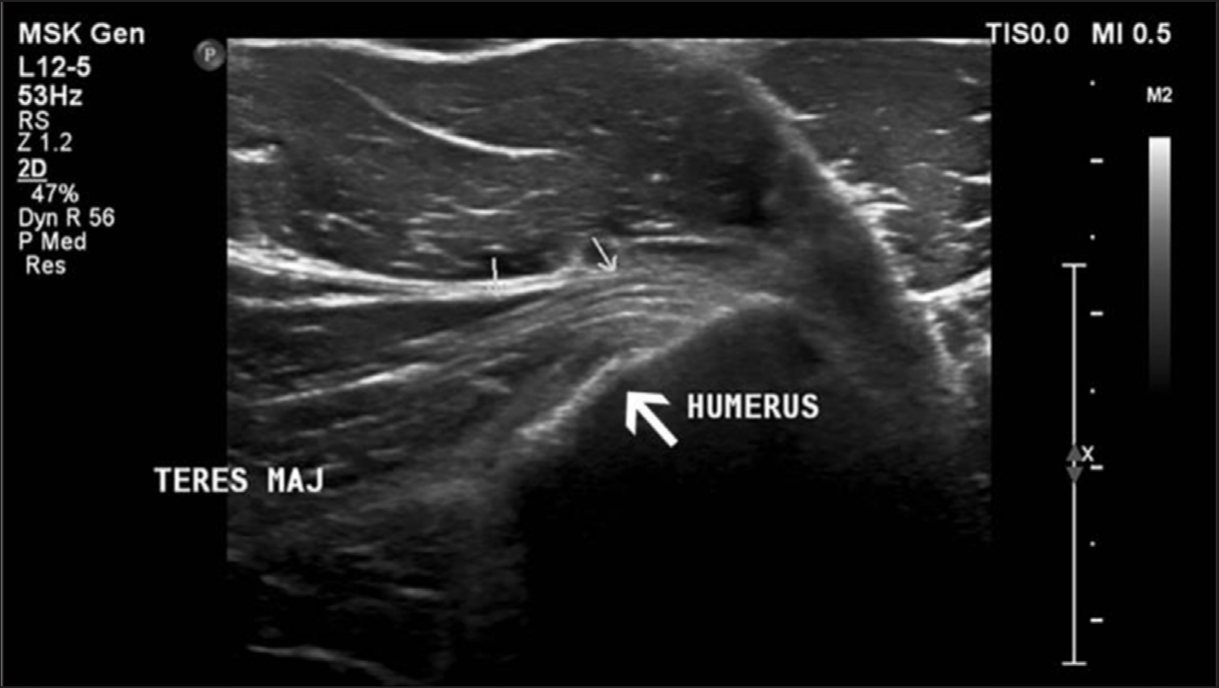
Ultrasound imaging (axial image) reveals an irregular delineation (large white arrow) of the teres major insertion at the medial aspect of the humerus posteromedial to the pectoralis major tendon (small arrows).
